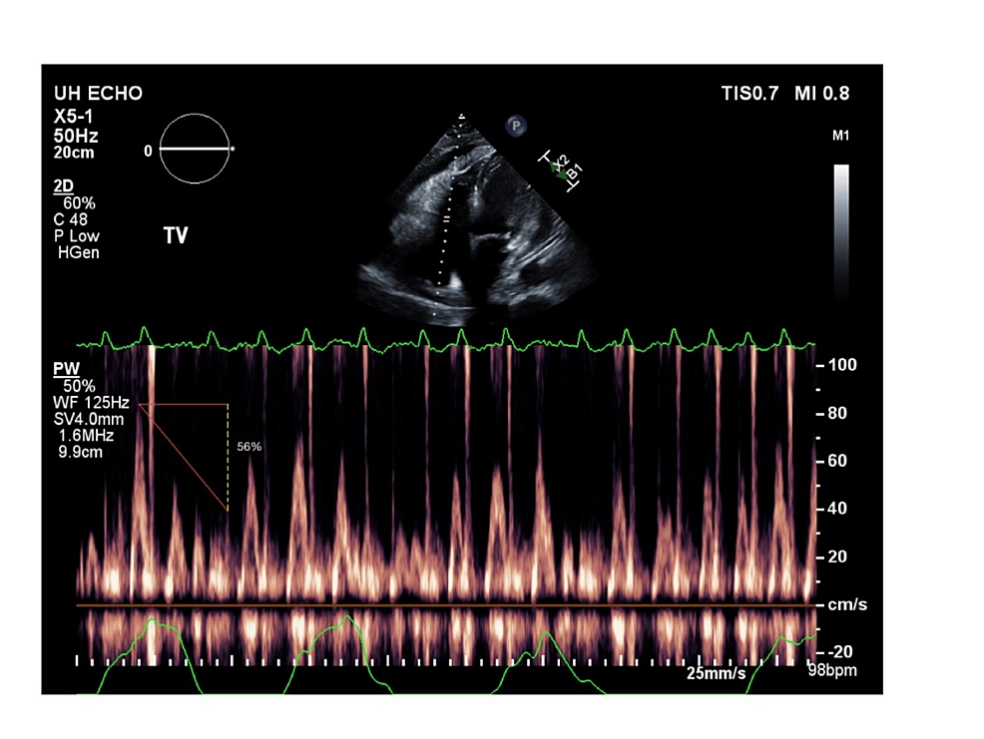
Continuous Doppler study showing the respiratory variation in tricuspid inflow velocity of 56% The peak E-wave velocity across the tricuspid valve will decrease by at least 40% in expiration compared to inspiration during cardiac tamponade [8].
